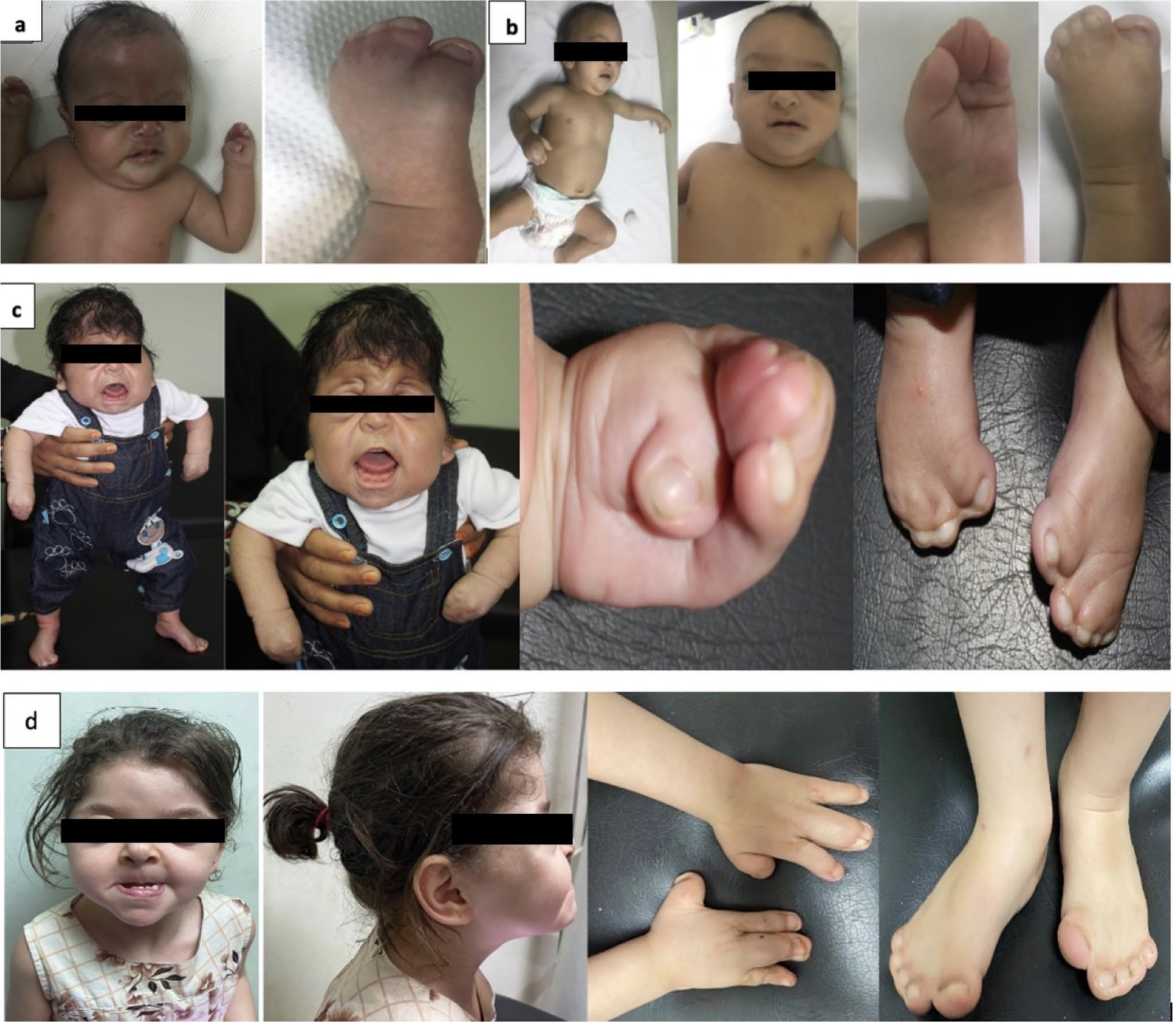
Clinical phenotype of patients: a: Patient 1 shows a broad forehead, frontal bossing, proptosis, depressed nasal bridge, long philtrum, syndactly, and broad foot. b: Patient 2 shows broad forehead, frontal bossing, proptosis, depressed nasal bridge, short philtrum, retrognathia, maxillary hypoplasia, syndactly, and broad hand and foot. c: Patient 4 shows depressed nasal bridge, hypertelorism, beaked nose, long philtrum, and syndactly of hand and feet. d: Patient 5 shows a broad forehead, proptosis, depressed nasal bridge, short philtrum, syndactly, and broad hands and feet. X-rays of the hand and feet shows bone syndactly
